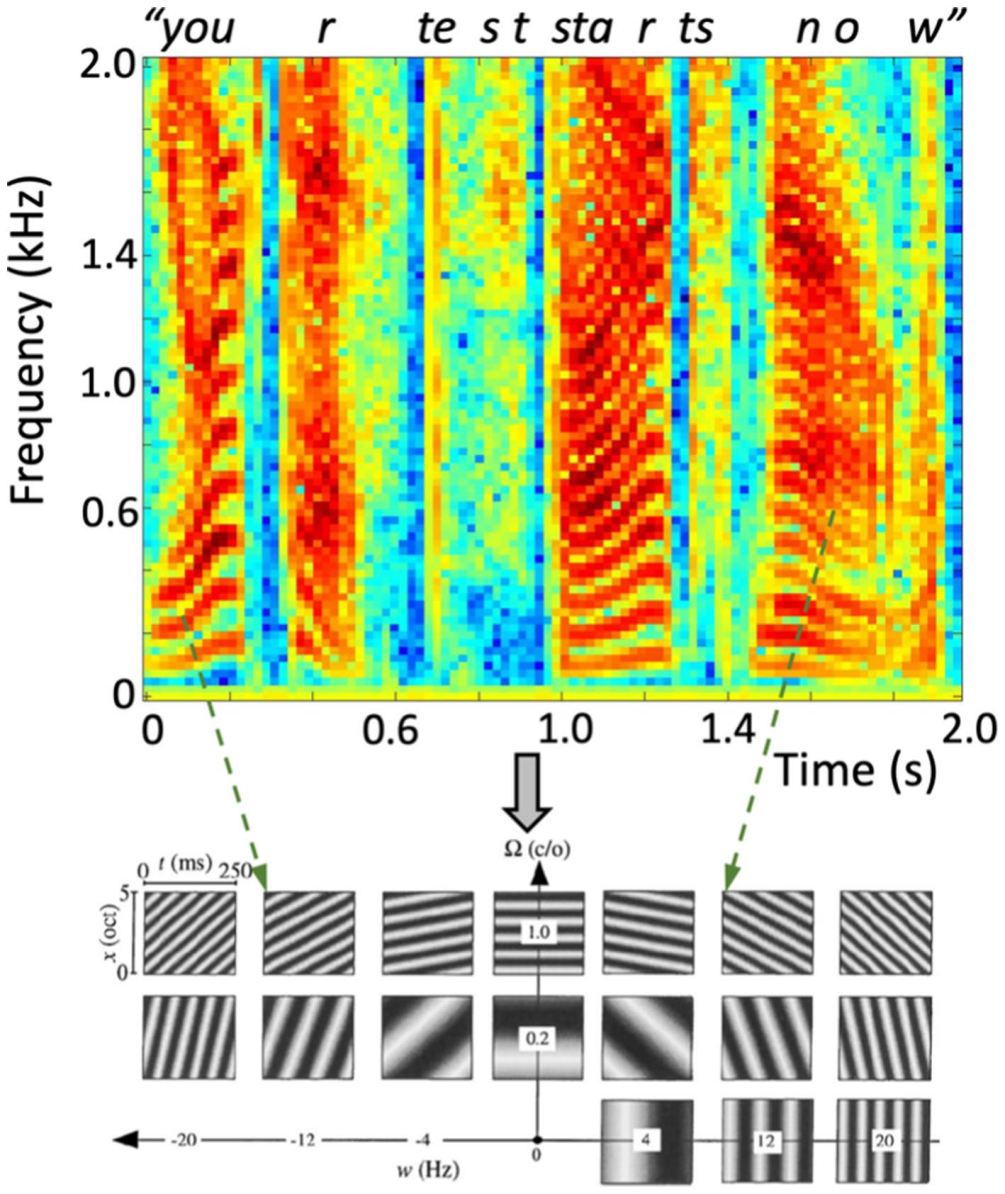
(Top) Spectrotemporal representation (sonogram) of the amplitude of a human speech utterance “Your Test Starts Now”, containing a complex dynamic sequence of rising and lowering harmonic complexes (the vowels, e.g., at 0–0.25 s, 1.0–1.2 s, 1.45–1.95 s), interspersed with broad-band nonharmonic fricatives (e.g., at 0.7, 1.35 s). (Bottom) Dynamic ST ripples can represent the full space of joint spectrotemporal modulations at any time-frequency interval. Here, 17 examples of 0.25 s with different densities and velocities are shown over the full bandwidth of 5 octaves. Two instances in the recorded speech signal point at roughly corresponding ripples (dashed arrows).
